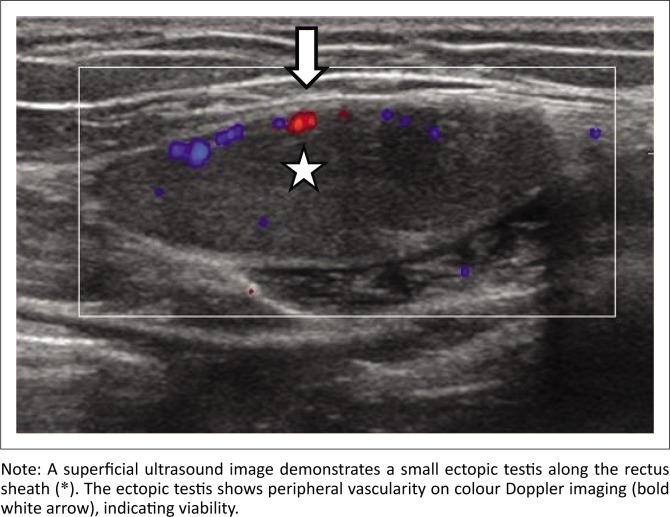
Sagittal ultrasound image of the left upper abdomen with a linear probe in a 2-year-old male child with left cryptorchidism.
